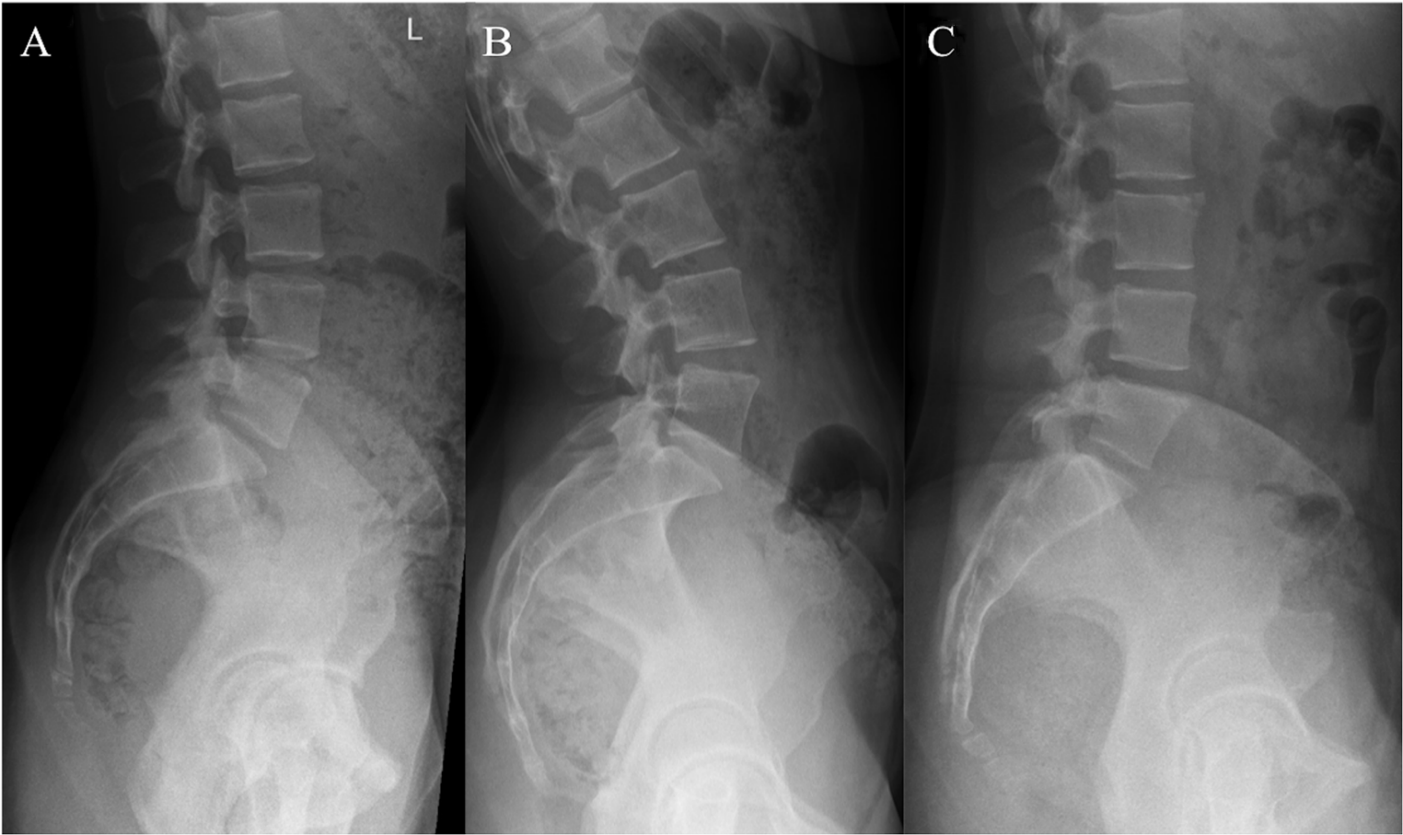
Representative radiographs with different types of static lumbopelvic alignment (A Normal – PI-LL -10° − 10°, B Hyperlordotic – PI-LL < -10°, C Flatback – PI-LL > 10°). PI pelvic incidence, LL lumbar lordosis
